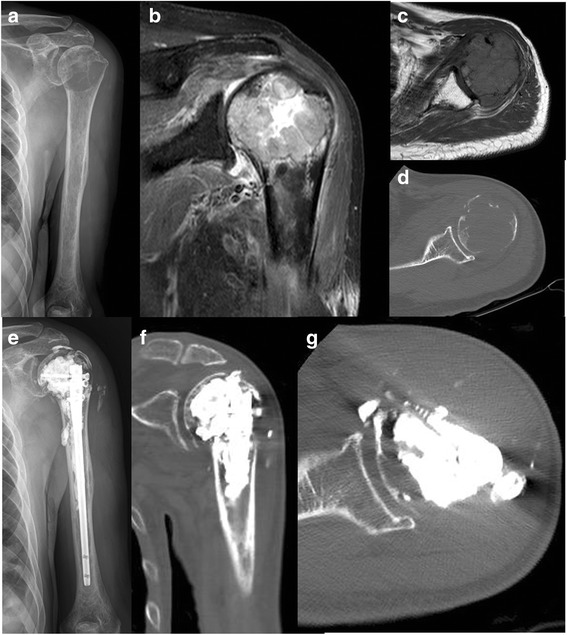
A case of a 62-year-old man with multiple lung, brain, and bone metastases due to advanced renal cell carcinoma. Preoperative plain radiograph (a), fat-suppressed enhanced coronal MRI (b), T1-weighted axial MRI (c), and CT (d) show osteolytic lesion in the humeral head. Postoperative evaluation reveals stable fixation by proximal self-locking screws of IM nail and PC in plain radiograph (e), coronal reconstructive CT (f), and axial CT (g)
